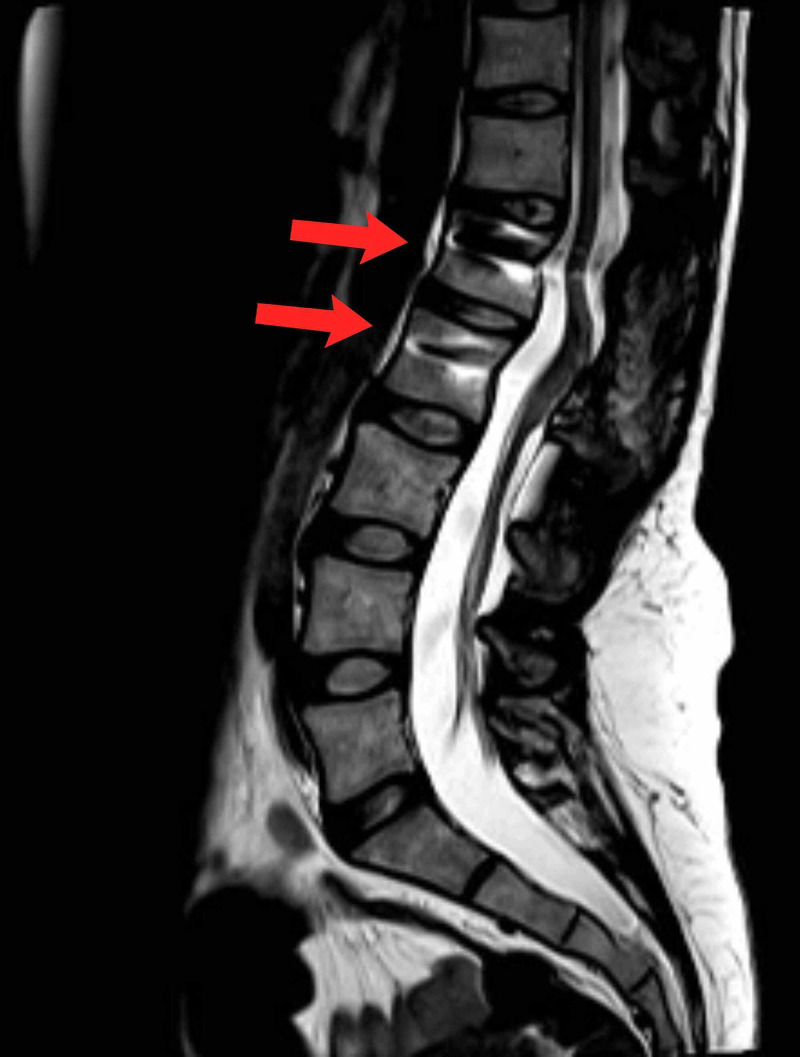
Sagital magnetic resonance imaging Postoperative T2 sagittal MRI scan at the levels L1 and L2.
